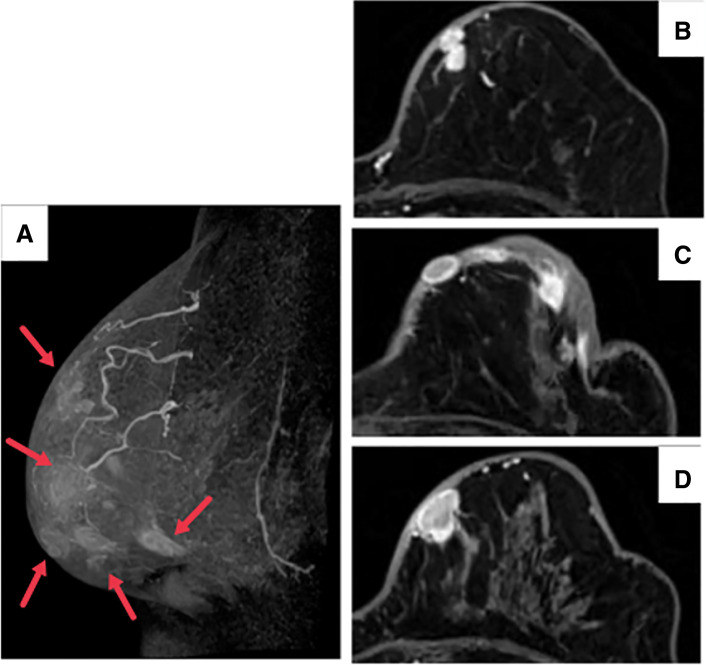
MRI findings. (A) Lateral 3D reconstruction showing multiple vascularized masses (red arrows). (B–D) Axial views demonstrating heterogeneous contrast enhancement.
